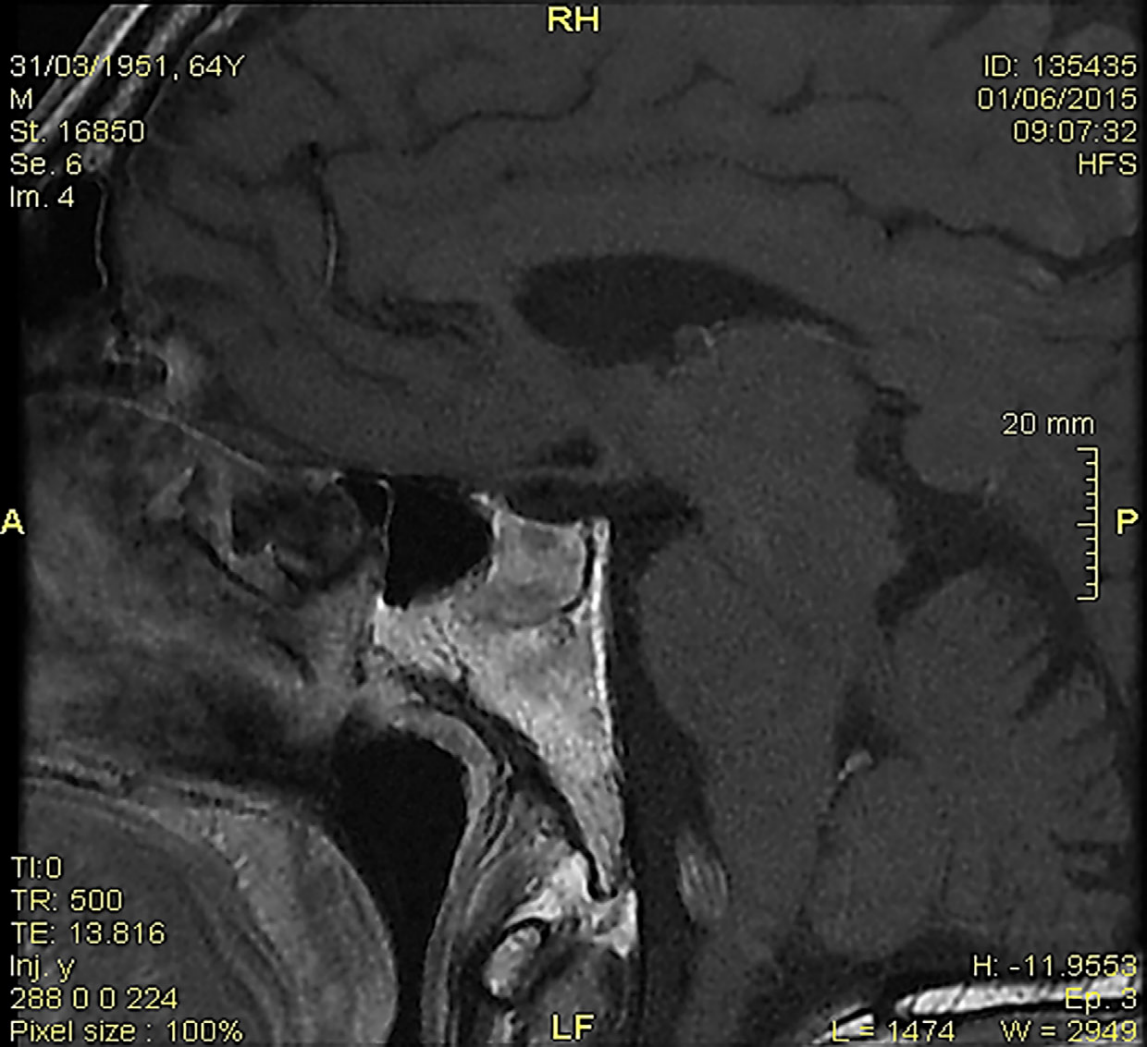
At the magnetic resonance imaging, pituitary microinfarcts in an enlarged gland were shown; the hypophyseal stalk was normal, and the neurohypophyseal ‘bright signal’ in T1 sequences was undetectable.
